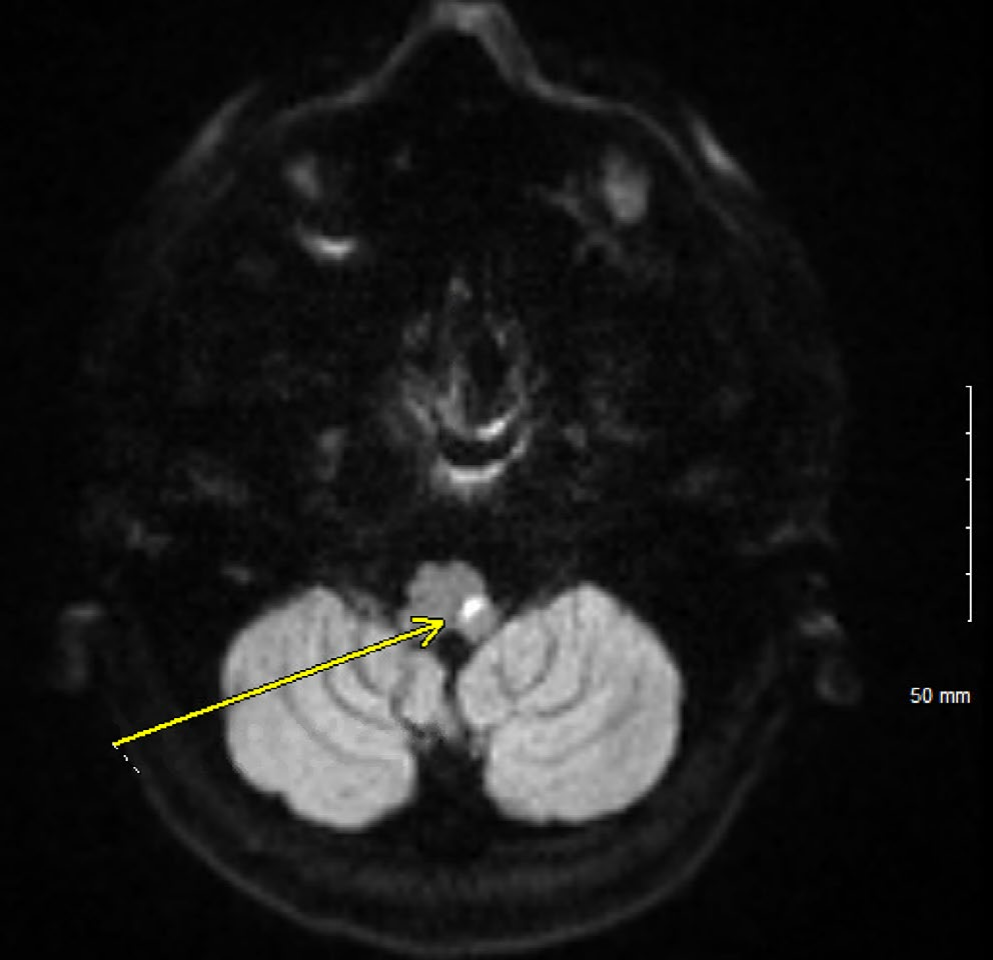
Increased DWI signal is seen in the medulla indicating acute infarction (yellow arrow)
